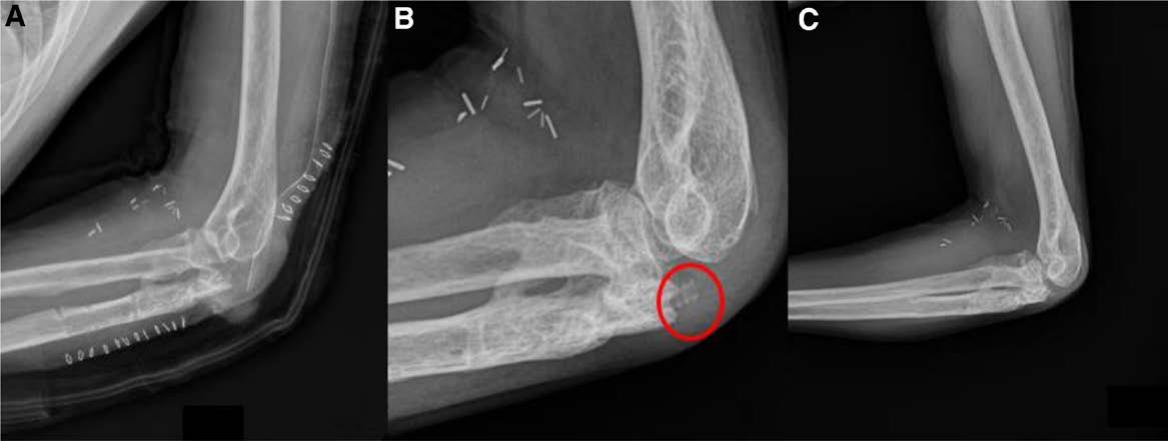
(A) Immediate postoperative X-ray. (B) POD 2 mo, protrusion of biocork screw was identified. (C) POD 7 mo, there was no more protrusion. POD = postoperative day.
